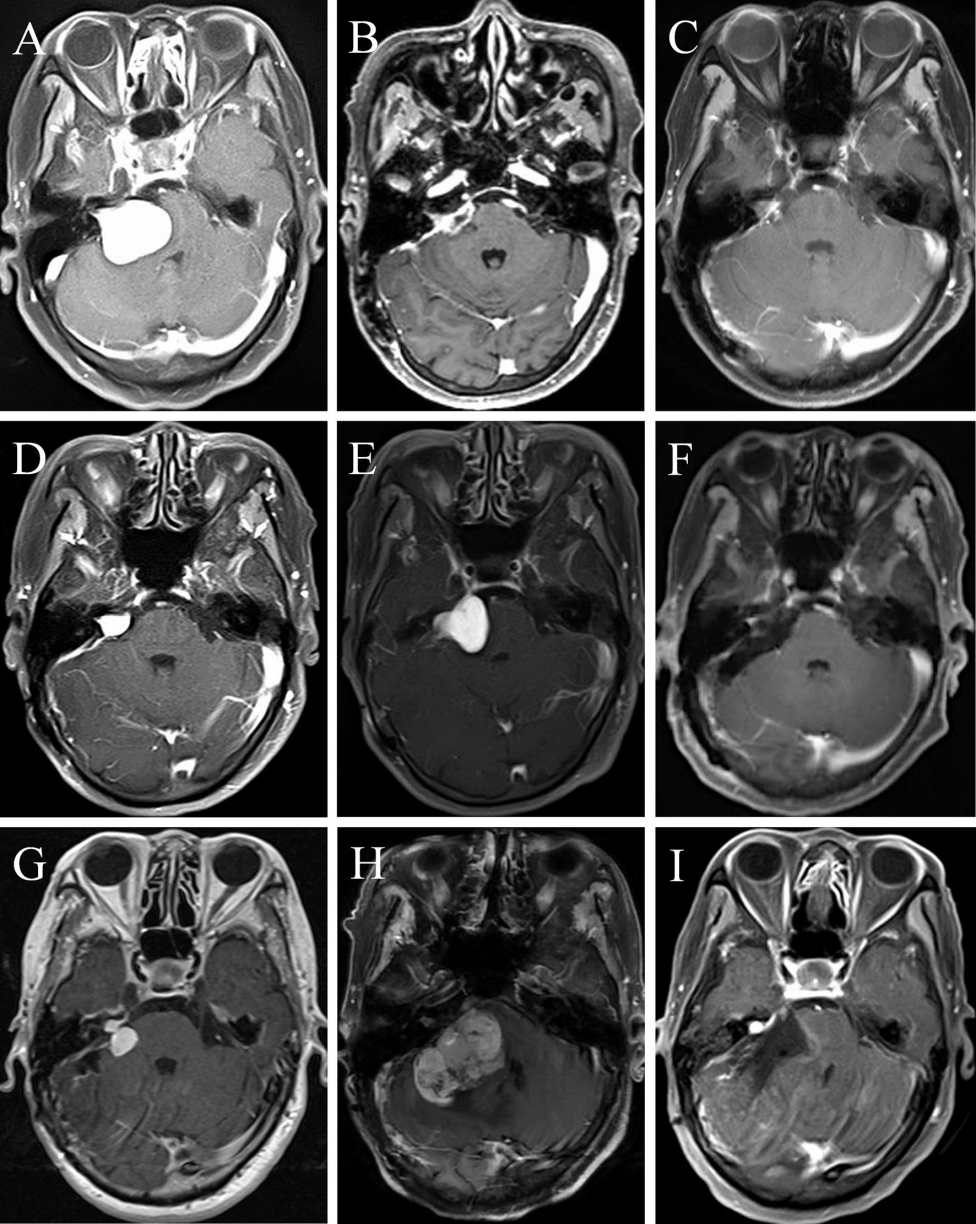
A female MTVS patient underwent multiple operations and radiotherapy with a 40 months’ survival time. Case 2. Preoperative contrast-enhanced magnetic resonance image (MRI) (A) of a 64-year-old female depicted a homogeneously enhanced tumor located at right cerebellopontine angle (CPA). After a NTR, enhanced MRI 3 months postoperatively (B) showed a remnant tumor and gamma knife radiosurgery (GKS) was conducted. Eight months after GKS, the tumor showed slight shrink on contrast-enhanced MRI (C). However, enhanced MRI 2 years (D) and 2.5 years (E) after operation demonstrated regrowth of the tumor. She underwent the second operation and the pathological diagnosis was MPNST (WHO grade 3/FNCLCC grade 2). Postoperative MRI (F) confirmed gross total resection (GTR). Two years later, the tumor regrew again and GKS was prescribed (G). One year later, the MPNST grew to a maximum size of 45mm with heterogenous enhancement (H). The patient received the third operation and postoperative MRI (I) demonstrated tiny remnant tumor located at the roof of internal auditory meatus.
